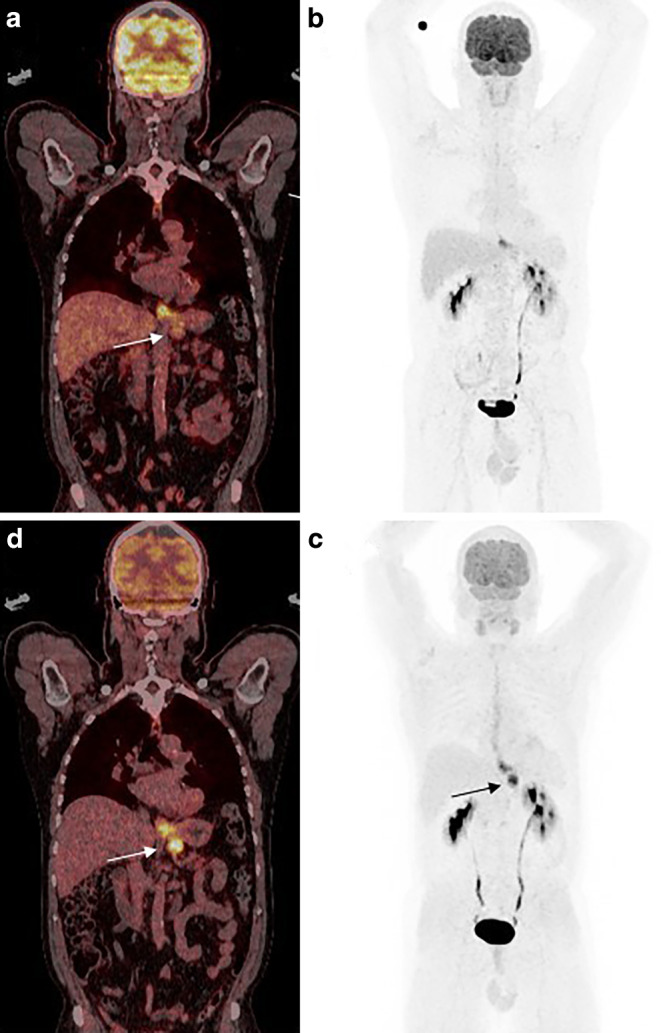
a Coronal section of a fused single-tracer [^18^F]FDG-PET/CT and maximum intensity projection (MIP) in (b) show a tracer uptake in the gastroesophageal junction with suspicious enlarged mesenterial lymph node without tracer accumulation (*arrow*). Tracer accumulation of the tumor and suspicious lymph node (*arrow*) could be visualized with dual-tracer PET/CT using [^18^F]FDG&[^68^Ga]Ga-FAPI-46 in fused images viewed in the coronal plane (c) or on MIP (d). Physiologic enhancement and liver are visible in both scans
